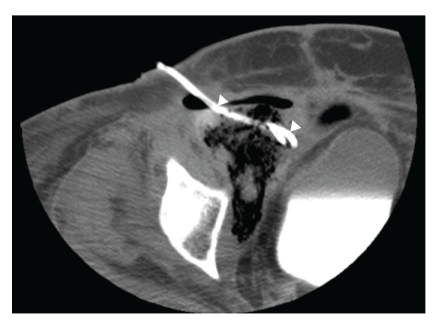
Noncontrast CT image following percutaneous drainage shows 12 French catheter (arrowheads) in anterior aspect of pelvic abscess.
